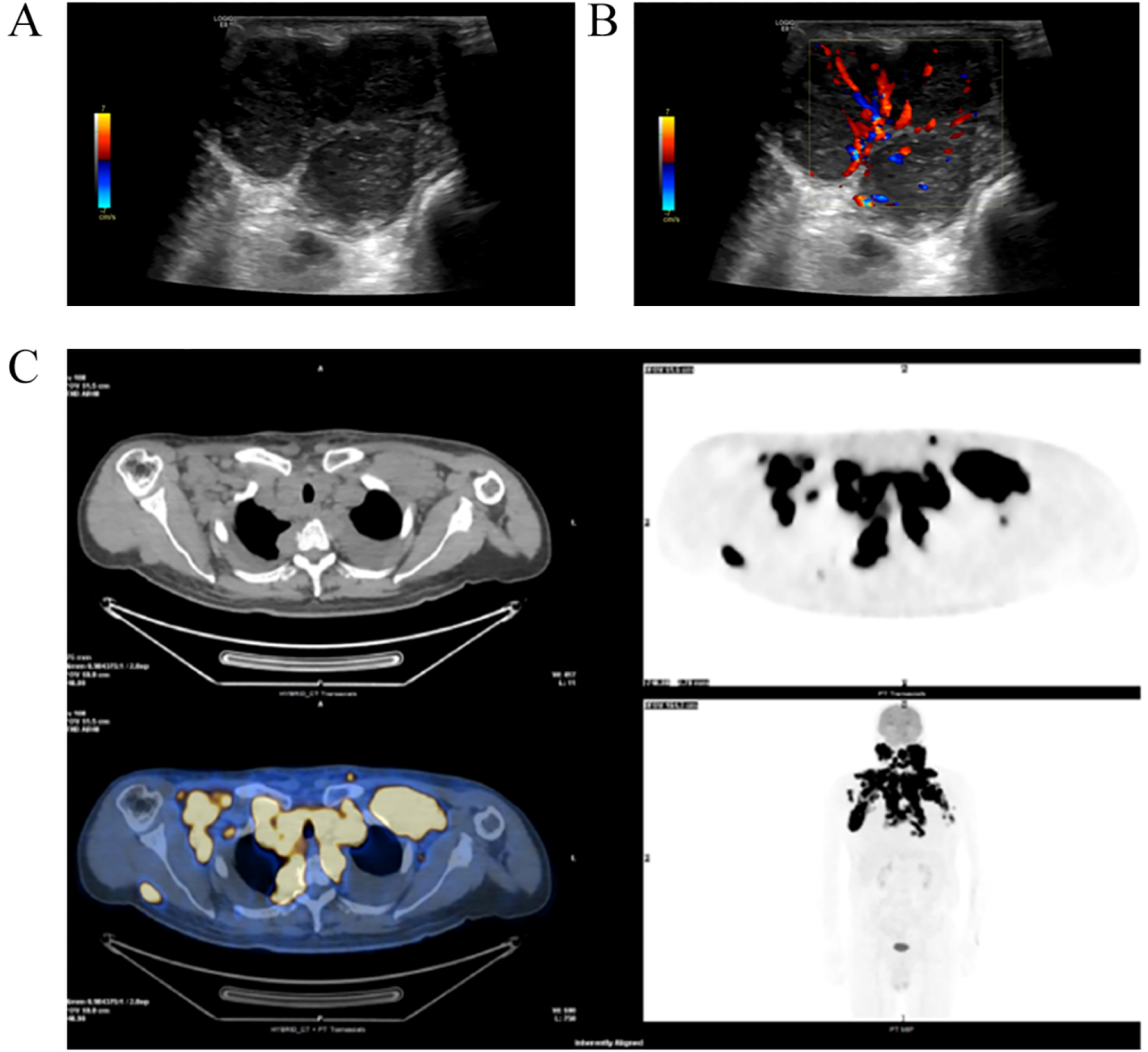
Representative baseline imaging features of DLBCL. (A) B-mode (2D ultrasound) image showing multiple fused hypoechoic nodal lesions with indistinct margins and absence of a visible hilum. (B) Color Doppler flow imaging (CDFI) demonstrating multiple strip-like intranodal blood flow signals, corresponding to Adler grade III vascularity. (C) PET/CT image revealing multiple hypermetabolic lymph nodes in the bilateral cervical, supraclavicular, and mediastinal regions, with a maximum standardized uptake value (SUVmax) of 24.5.
